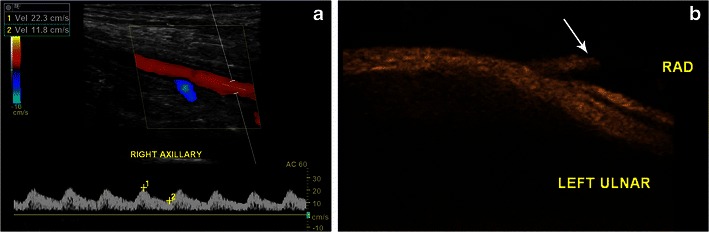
Arterial vascular obstruction. Spectral Doppler image of the right axillary artery (a) in a 42-year-old female on VA ECMO demonstrates diminished amplitude and prolonged systolic peaks of the arterial waveforms, classic for pulsus parvus et tardus. In a different patient, B flow ultrasound (b) shows complete occlusion of the radial artery (*arrow*) just distal to the bifurcation
